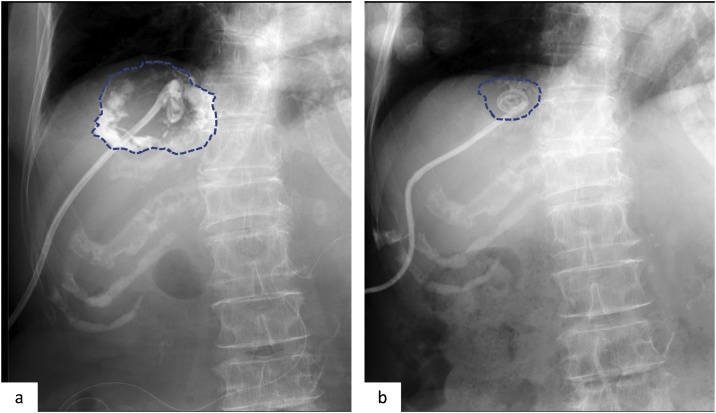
Sclerotherapy using minocycline hydrochloride under radiography. The dotted line indicates the target cyst. a. Initial sclerotherapy. Minocycline hydrochloride (200 mg) dissolved in 20 cc of saline is injected through the catheter. b. Before catheter removal. The cyst is greatly decreased in size compared to that after the initial sclerotherapy.
